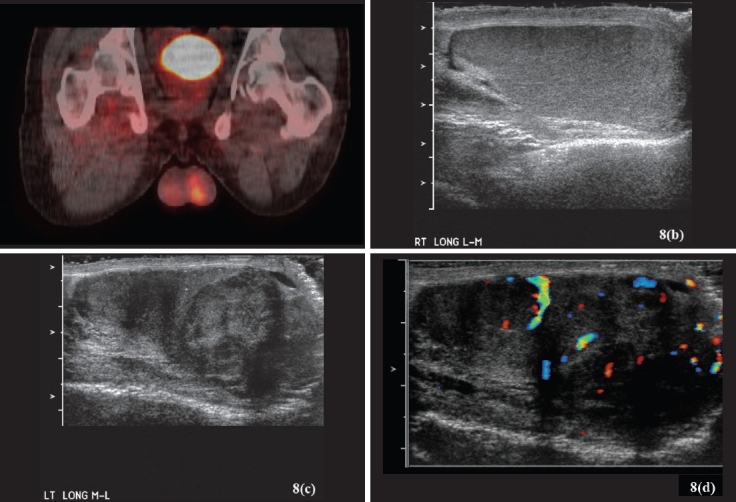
PET scan and scrotal US. Patient is a 19-year-old male with cerebral palsy and a history of neurofibromatosis. He underwent a PET scan to evaluate a retroperitoneal mass. The PET scan(a) demonstrated increased uptake by the left testis (the increased area of signal in the pelvis is the urinary bladder that lights up as the tracer is excreted). Physical examination and a scrotal US (b-d) demonstrated a left testicular mass. The patient underwent a left radical orchidectomy and was found to a have Stage 1 seminoma. (a) A maximum intensity anterior projection image of a PET scan with 2 deoxy-2-fluoro-D-Glucose (FDG) demonstrating area of increased activity in the left testis. (b-d) Scrotal US images demonstrating normal homogenous appearance of the right testis(b). The left testis demonstrates loss of homogenicity and a discrete mass with normal blood flow pattern in the lower pole region(c-d)
